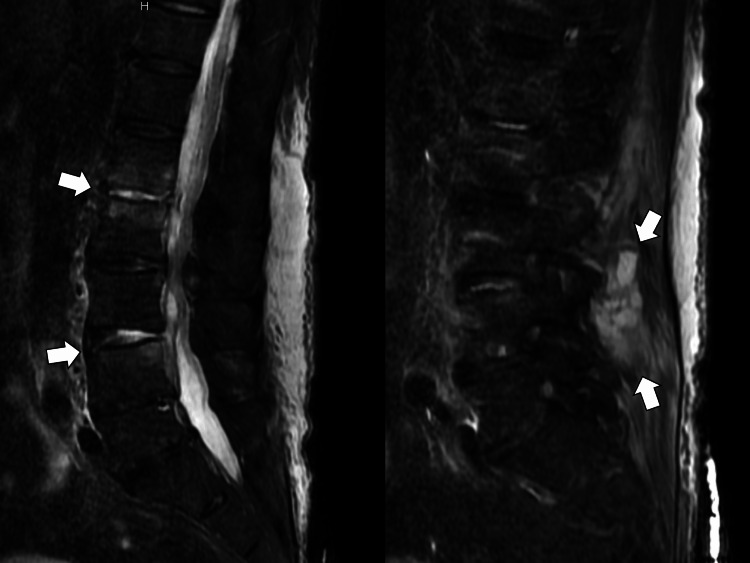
Magnetic resonance imaging (short tau inversion recovery) showing multiple vertebritis and paraspinal abscesses (white arrows)
